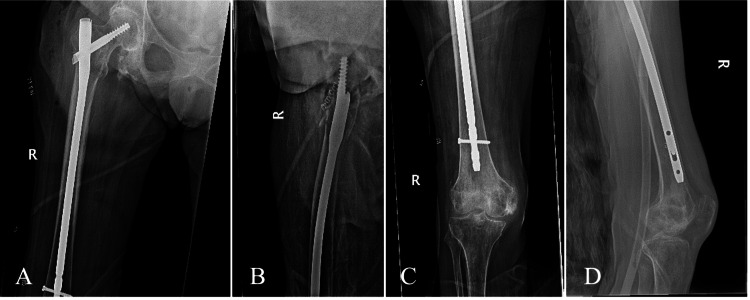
Immediate postoperative radiographs of the right femur demonstrating fixation of the right IT femur fracture with an unreamed intramedullary long nail A) Anteroposterior view of the right proximal femur. B) Lateral view of the right proximal femur. C) Anteroposterior view of the right distal femur. D) Lateral view of the right distal femur. IT: Intertrochanteric
